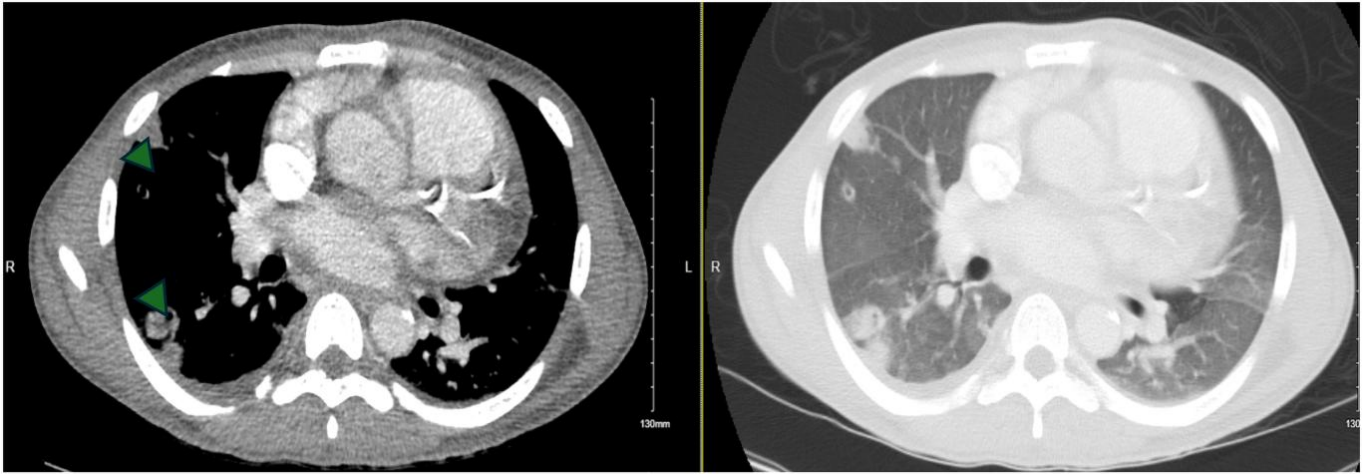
Computed tomography chest (axial slices) showing bilateral cavitary lung nodules (arrowheads) and ground glass opacities.
